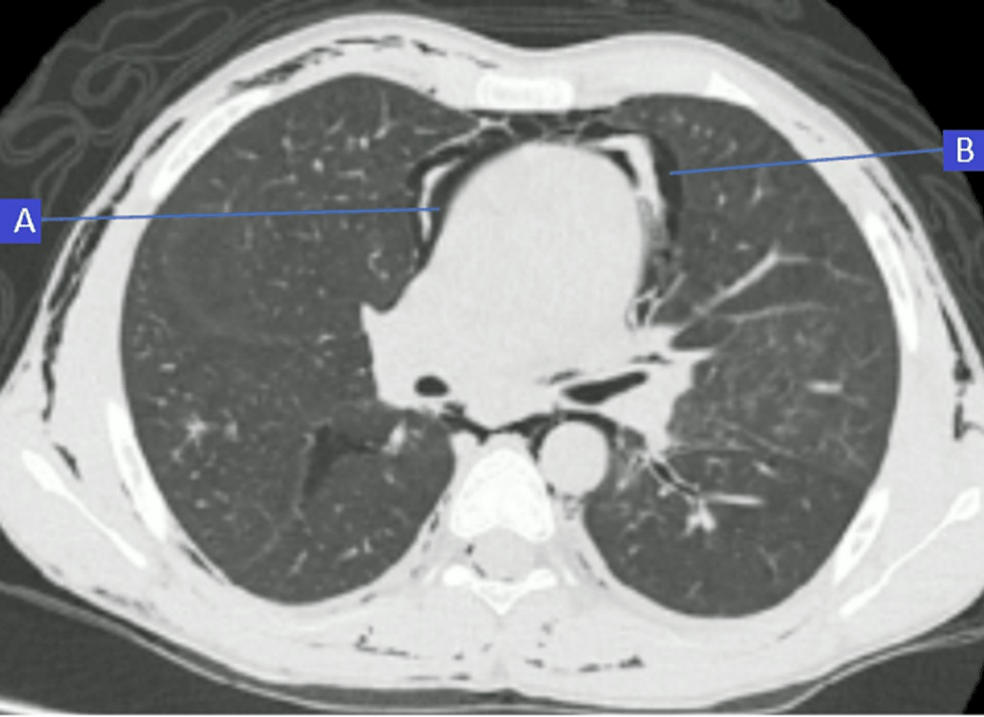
HRCT axial image below the level of carina Pneumopericadium (arrow A) and pnemomediastinum (arrow B)
